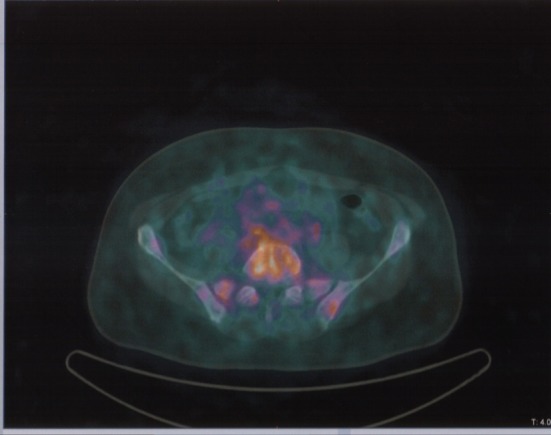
Same patient and acquisition parameters of Fig. 3. ^68^Ga-DOTANOC transaxial PET/CT at the level of fourth lumbar vertebra showing increased uptake of the radiopharmaceutical in a mixed metastasis.
